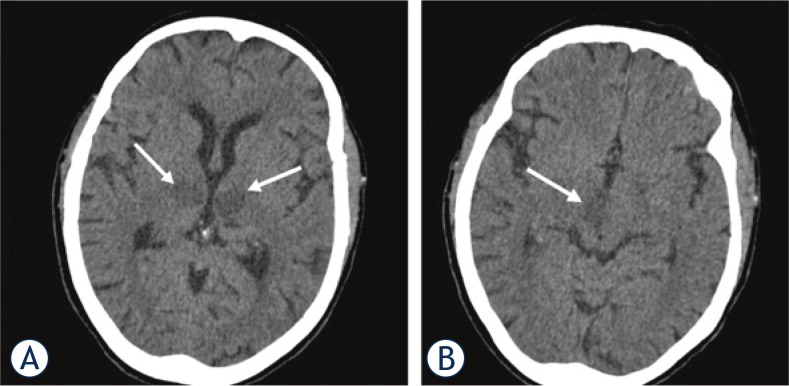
Another non-contrast head CT scan was performed 24 hours later and showed symmetrical ill-defined areas of hypodensity in the medial part of both thalami, corresponding to occlusion of the artery of Percheron (white arrows) (A). The hypodense area in the right thalamus extended into the anterior part of the mesencephalon and cerebral peduncle. The hypodense area in the left thalamus extended only into the anterior part of the mesencephalon (white arrow) (B).
